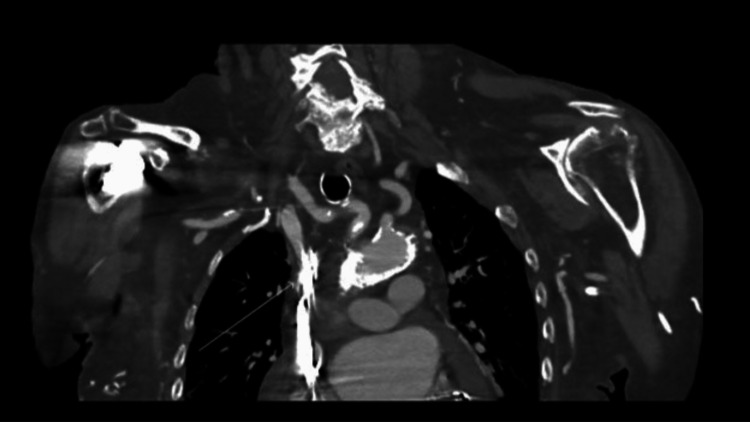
Computed tomography with intravenous contrast of the chest in the coronal plane showing the point of superior vena cava obstruction and a conglomeration of functional and abandoned pacemaker wires (white arrow).
